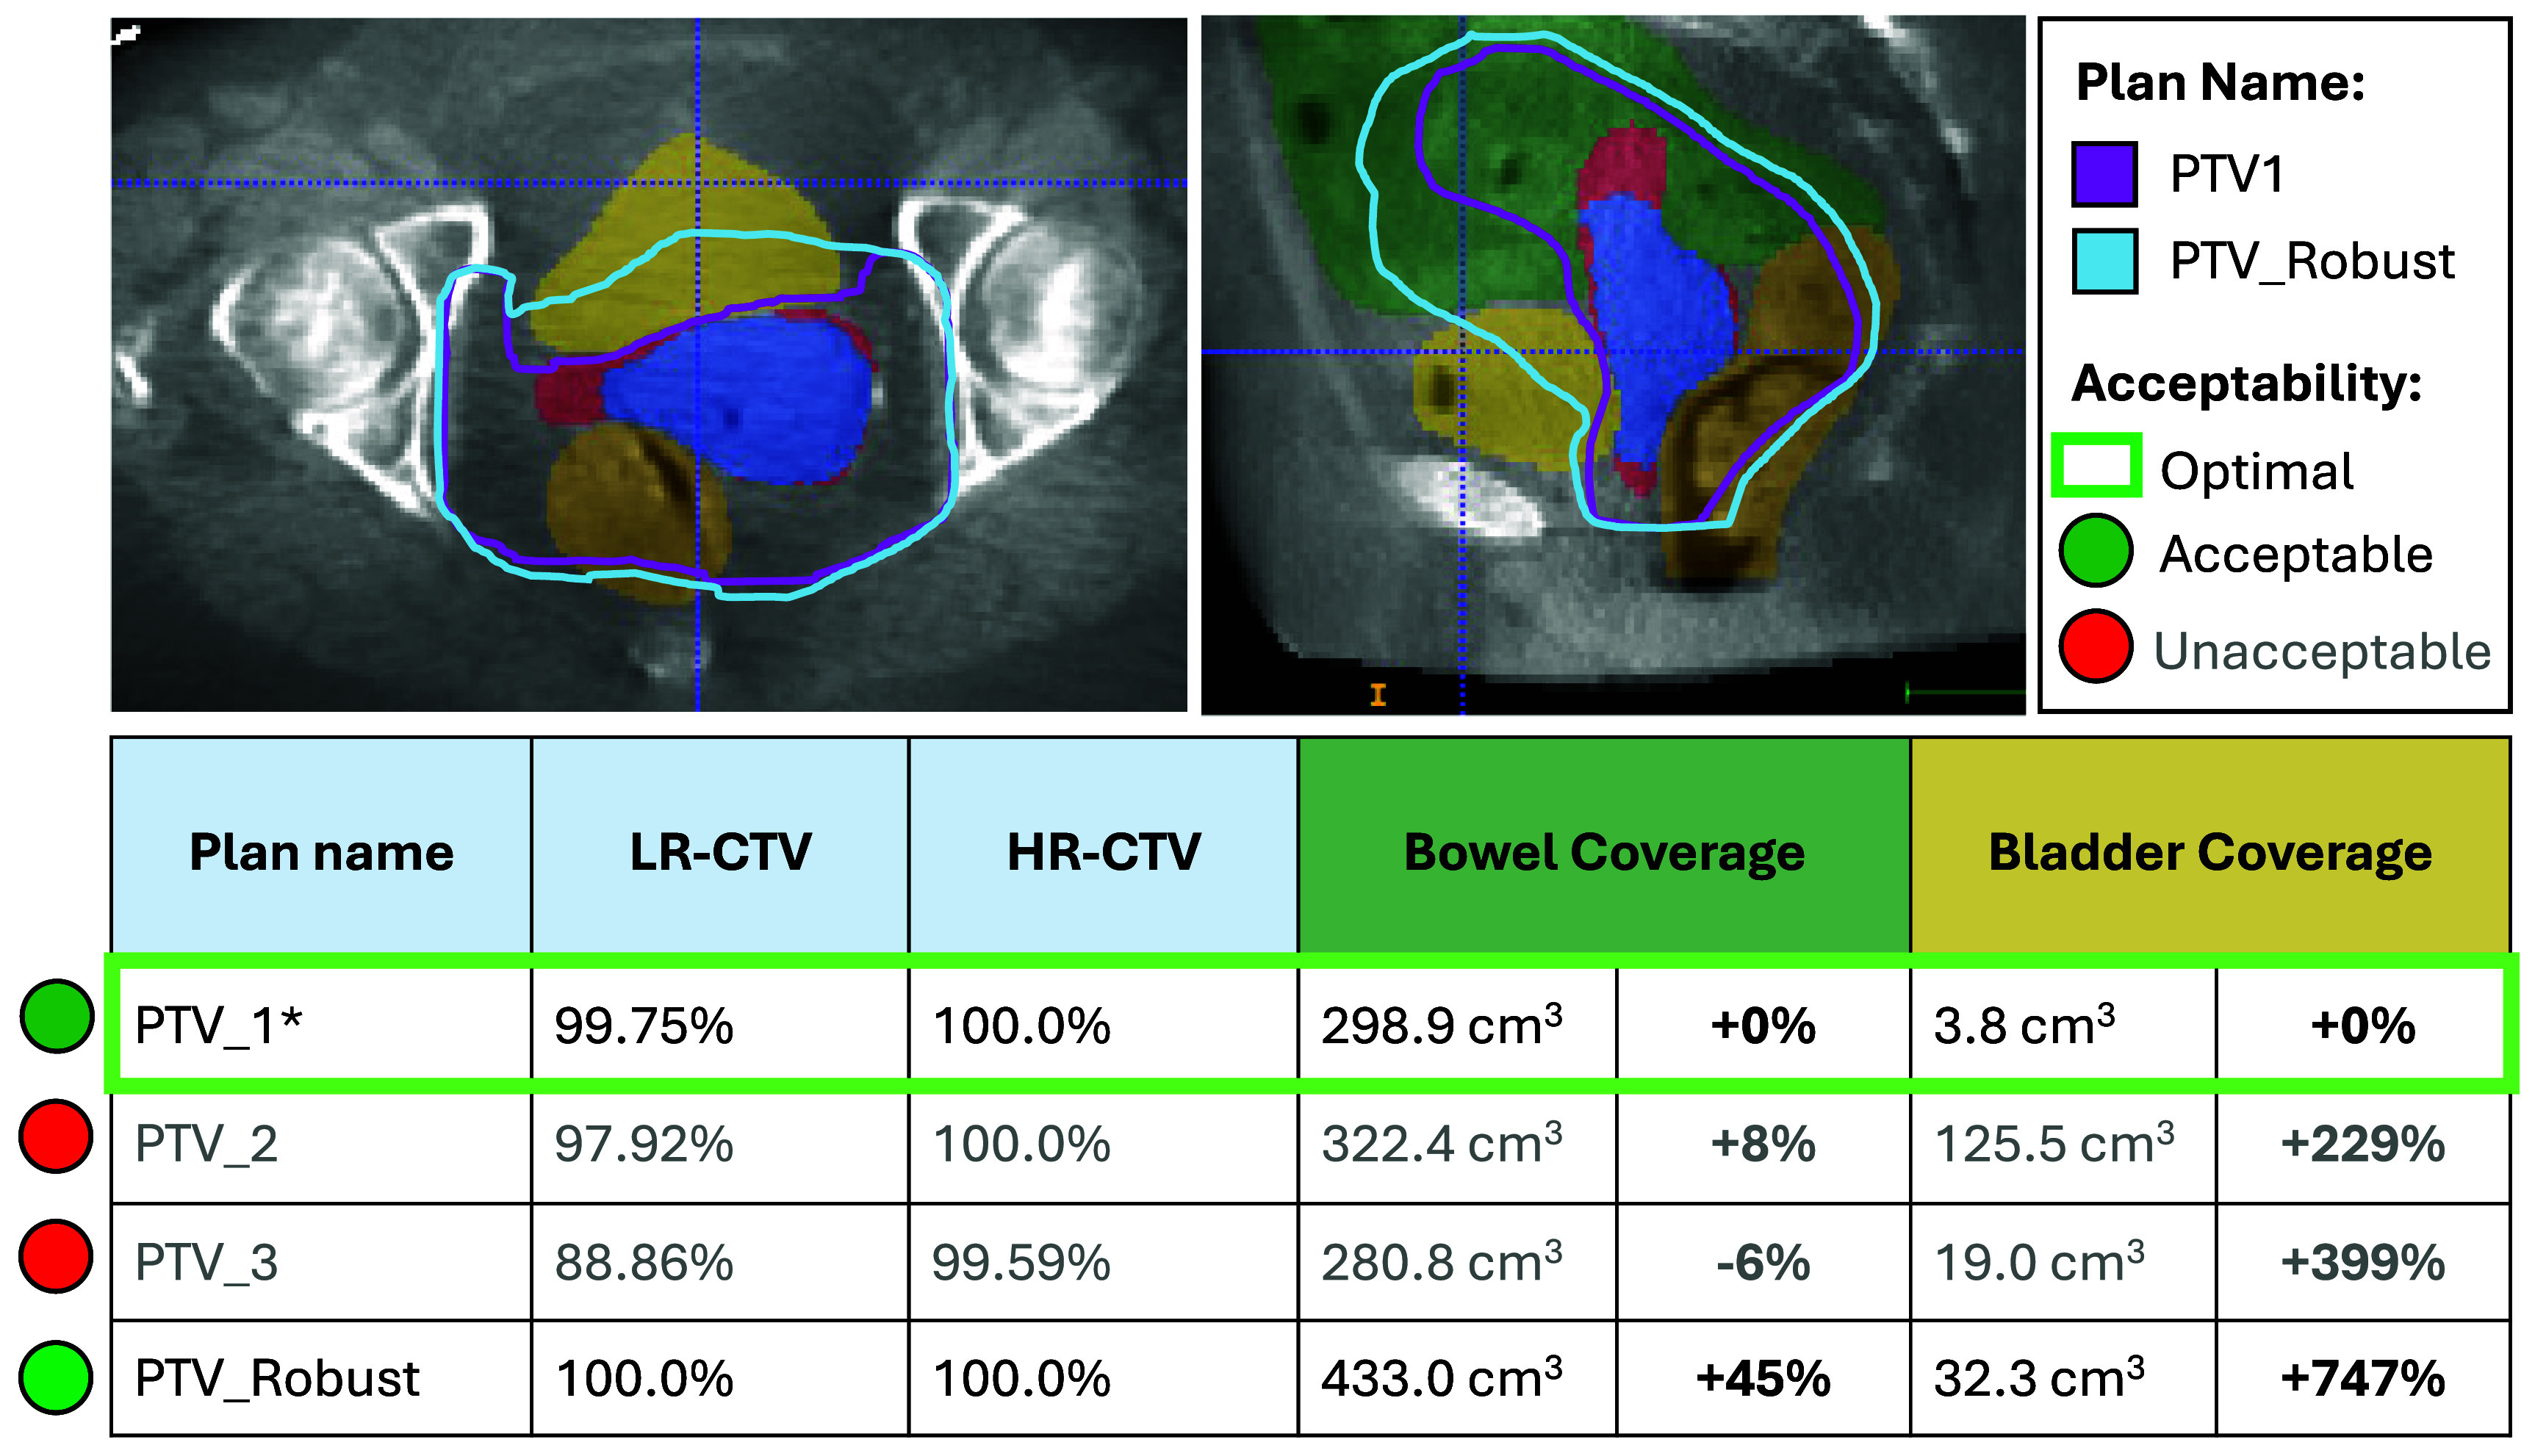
Mock-up of POTD assessment tool. All PTVs in the library and the underlying U-Seg3 segmentations are available for visual inspection on the 3D CBCT for transparency. (Note, only PTV1 and PTV_Robust are shown here for clarity). Target coverage is assessed according to the qSOP to flag plans as either ‘acceptable’ (green circles) or ‘unacceptable’ (red circles). The optimal plan indicated by the qSOP is highlighted by the green rectangle. Calculated values of LR-CTV, and HR-CTV coverage are given as a per cent for each PTV in the library. Similarly, the per cent difference in volumes for the bowel and bladder are also given (normalised to the acceptable plan with the minimum amount of bowel coverage).
